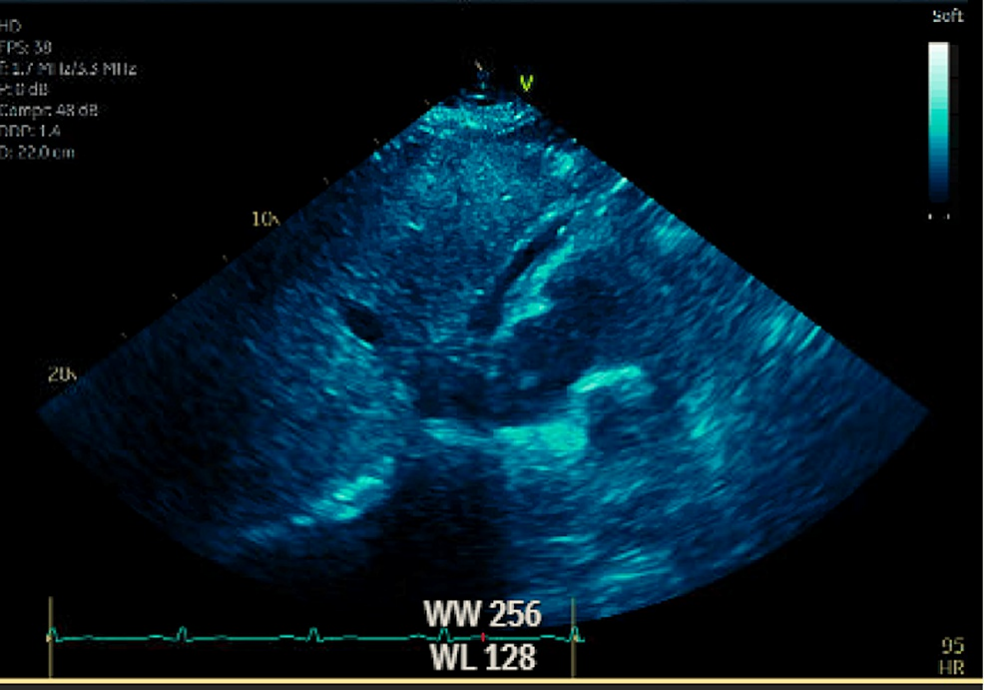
TTE subcostal view showing small anterior pericardial effusion TTE: Transthoracic echocardiography
